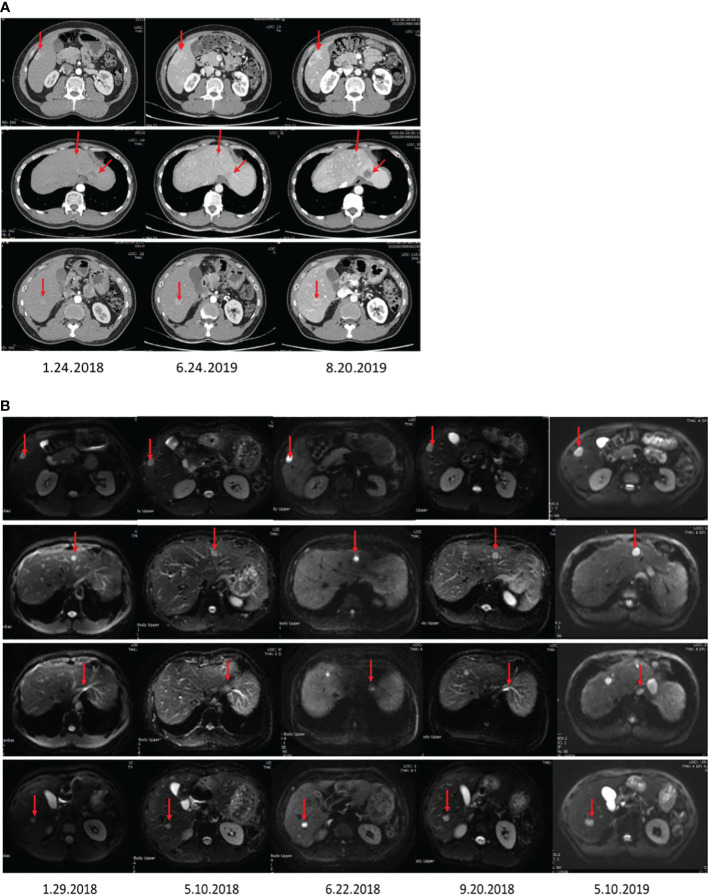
The images display the abdominal CT (A) and MRI (B) before surgery, showing multiple liver nodules.
